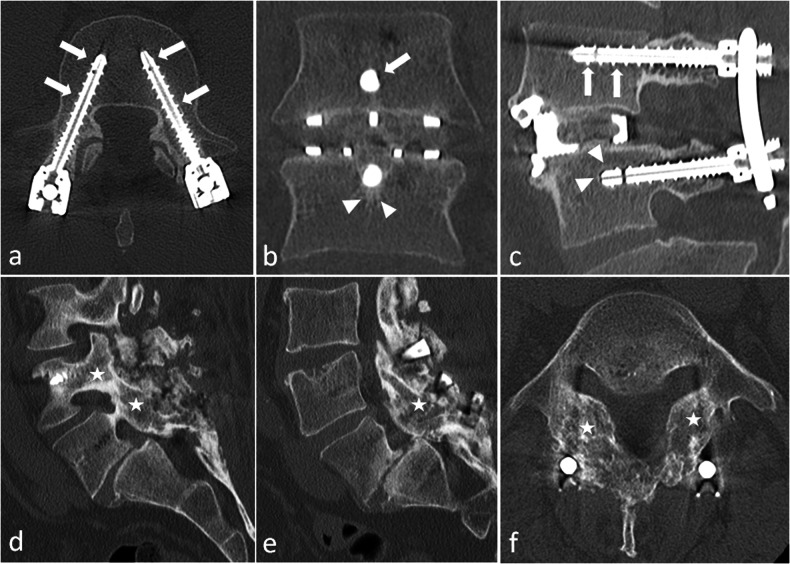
Normal aspect of an anterior and posterior lumbar intervertebral fusion, one year after surgery. On axial (a), coronal (b) and sagittal (c) CT images, no bone reaction, whether resorption or sclerosis, is seen around the screws (arrows), except a mild, thin sclerosis around the inferior screw on (c), which can be tolerated (arrowheads). Normal bone fusion in another patient. Sagittal (d, e) and axial (f) images show a full bony bridge on posterior columns, with cortical and trabecular continuity (stars). Also, no vacuum phenomenon (gas) is visible within the disks or around the interbody spacer
